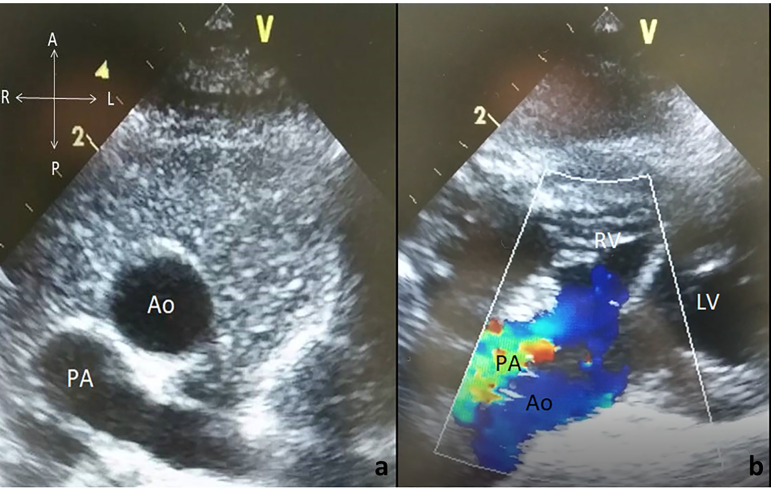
Echocardiographic two-dimensional image demonstrating the spatial relations of the great arteries in modified parasternal short axis view (a); color Doppler image showing that both great arteries are emerging from the right ventricle in modified subcostal view (b). Ao=aorta; LV=left ventricle; PA=pulmonary artery; RV=right ventricle
